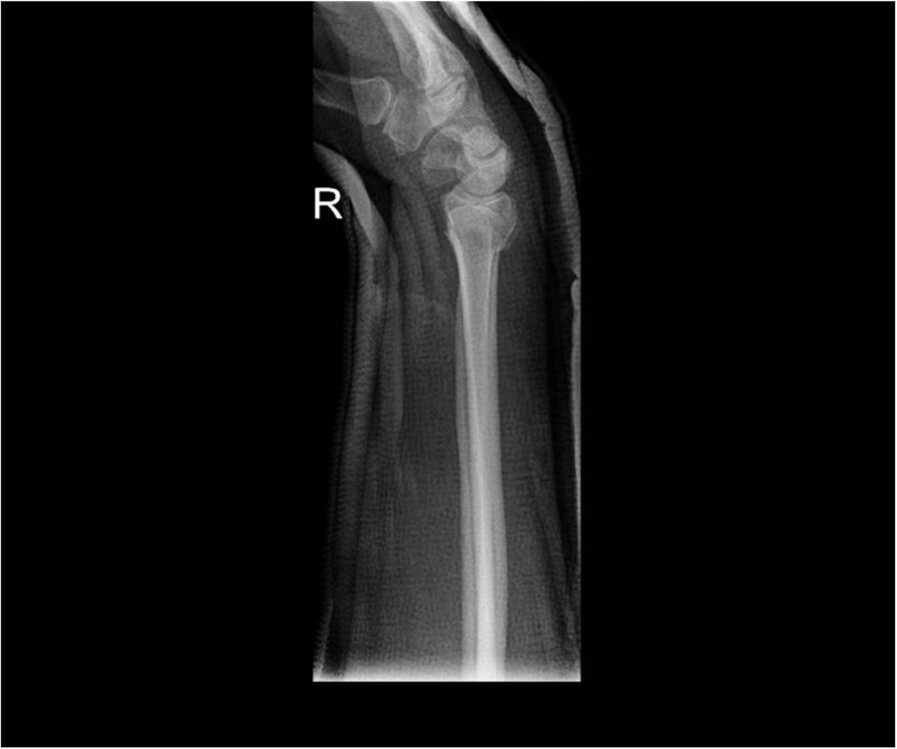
Pre- and post-manipulation radiographs of a 33-year-old lady with a right distal radius fracture
